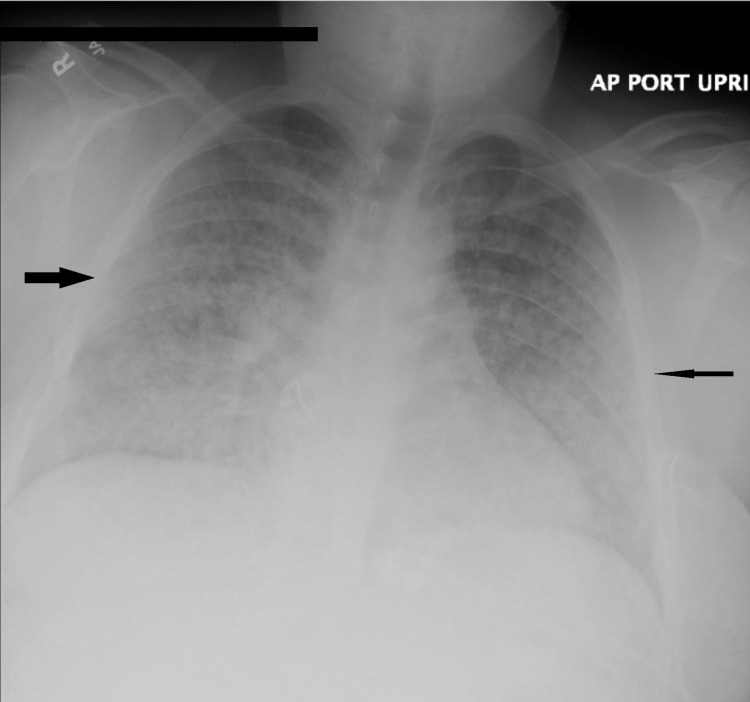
Chest X-ray shows diffuse pulmonary infiltrates (arrows)
